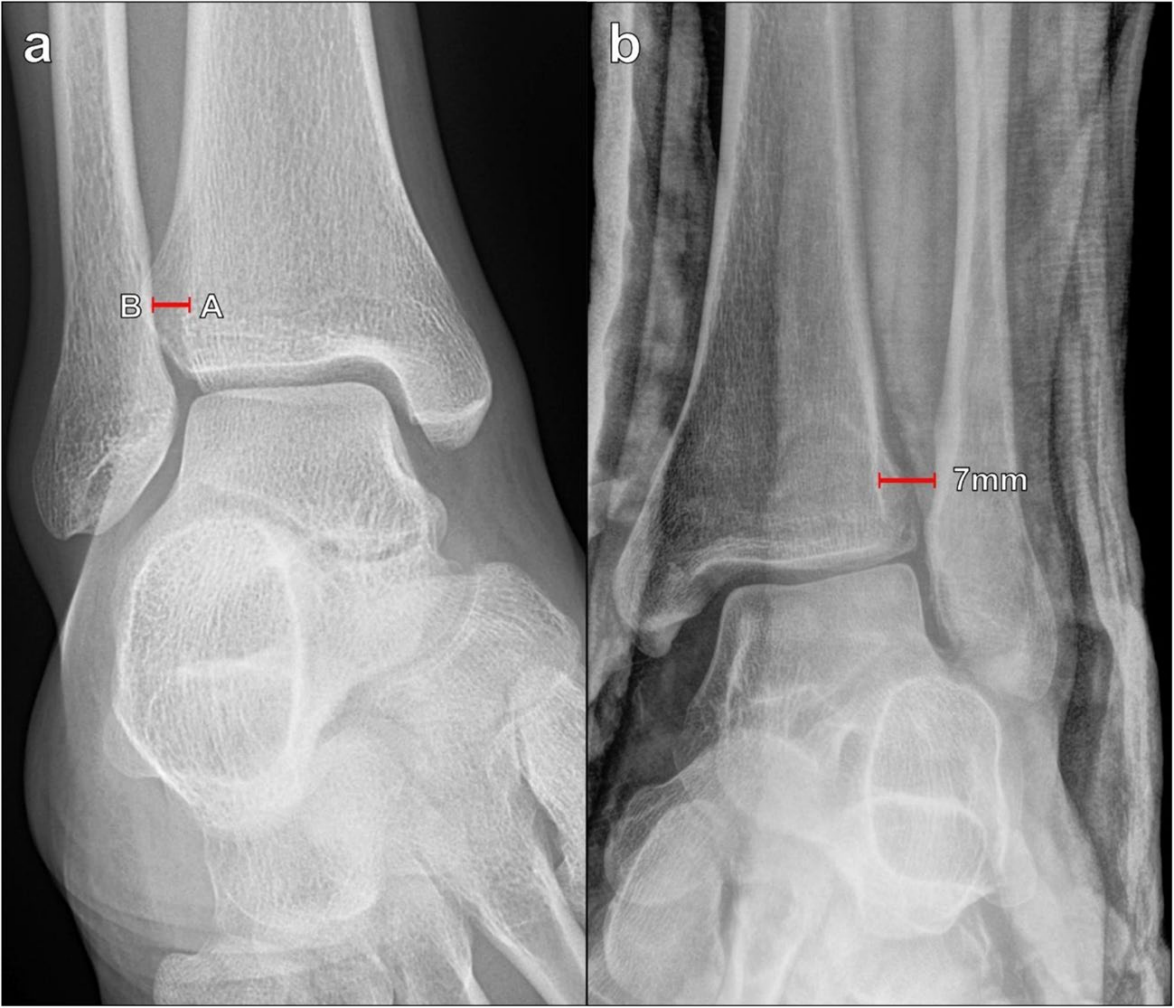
Measurement of the tibiofibular clear space on mortise radiograph of the ankle. a The measurement is made between the distal tibial incisura fibularis (A) to the medial margin of the distal fibula (B) at a level 1 cm superior to the tibial plafond. The normal tibiofibular clear space measures < 6 mm, and a measurement ≥ 6 mm indicates syndesmotic disruption. b Widening of the tibiofibular clear space on mortise radiograph of the ankle in a 20-year-old male patient after a fall. The tibiofibular distance measures 7 mm, and there is widening of the medial tibiotalar clear space, indicating deltoid ligament insufficiency as well
